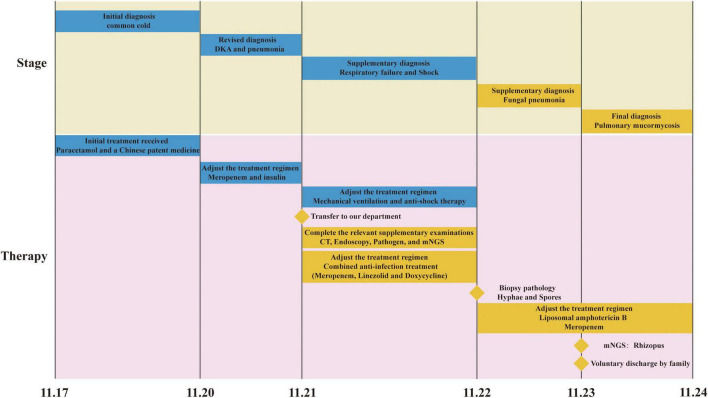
Timeline of the clinical course. Blue: outpatient/external diagnosis & treatment; yellow: in-hospital diagnosis and treatment. Key events from the initial common cold (11/17) to final diagnosis of pulmonary mucormycosis and voluntary discharge (11/24) are shown. CT Computed Tomography; DKA Diabetic Ketoacidosis; mNGS metagenomic Next-Generation Sequencing.
